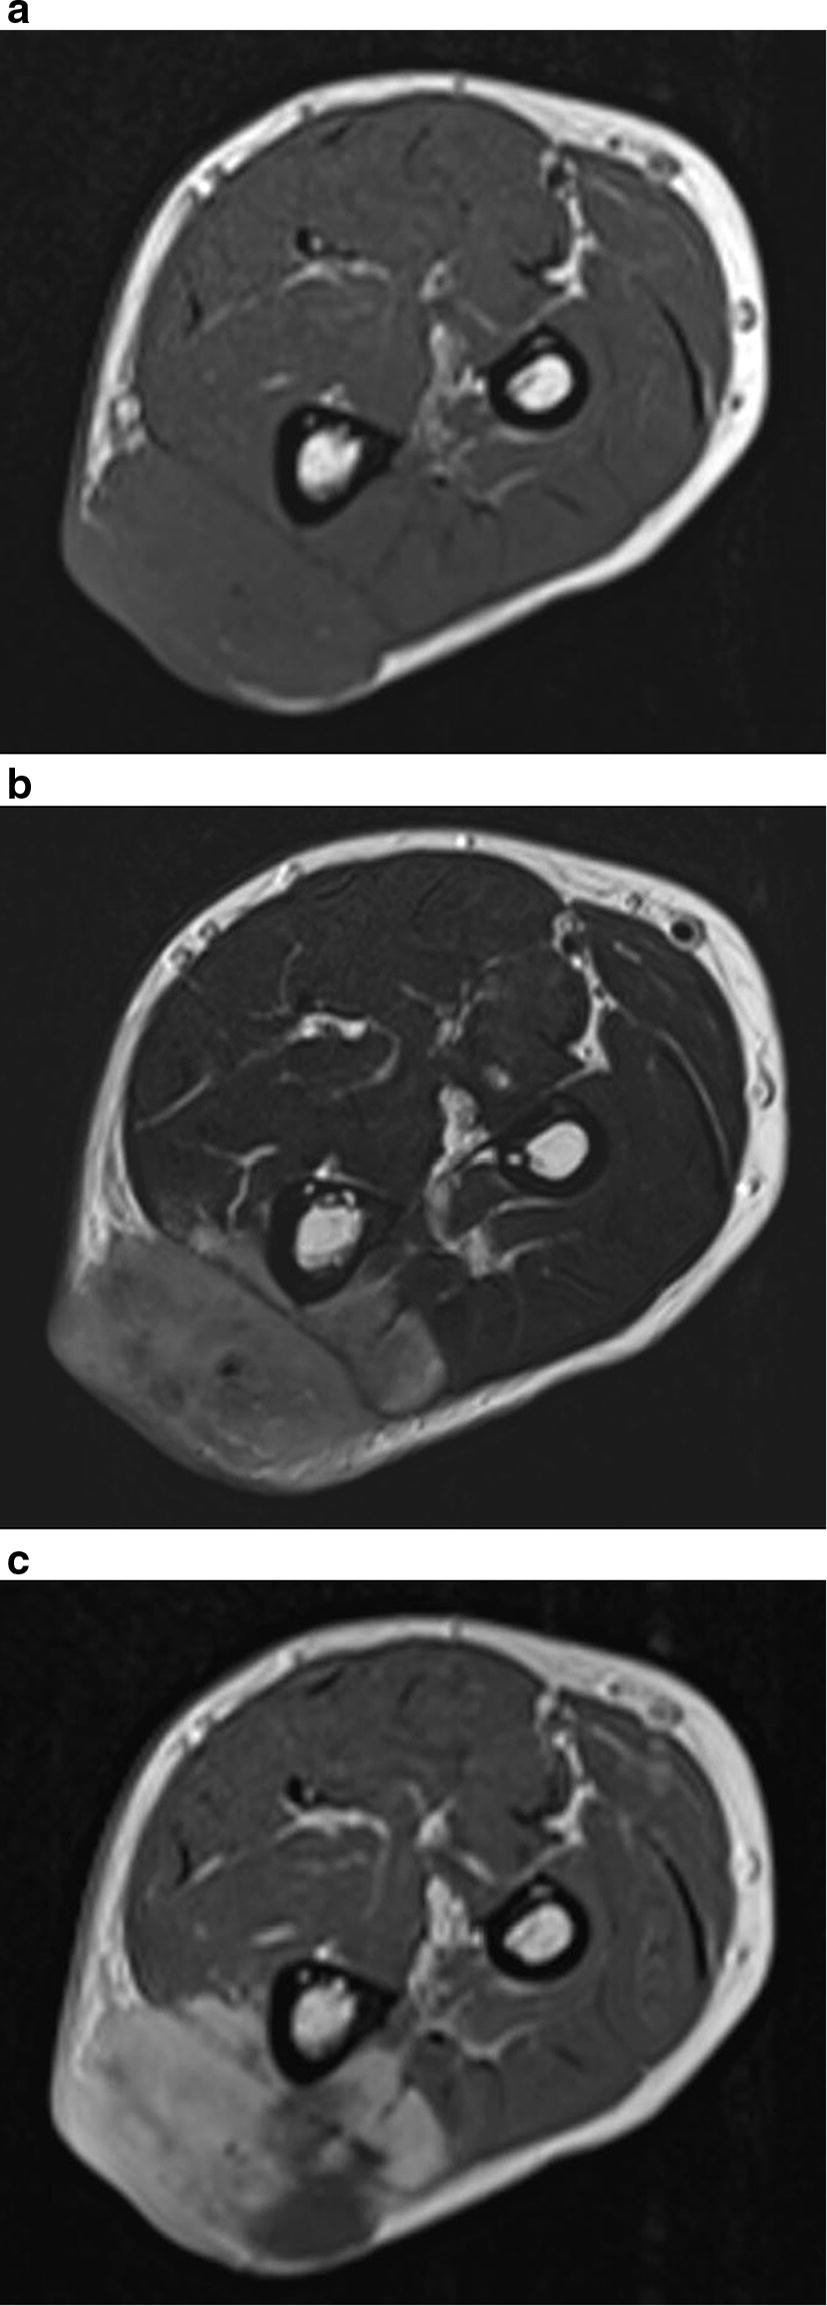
Magnetic Resonance Imaging (MRI) showing a soft tissue mass in the subcutis. The lesion is isointense to muscle in T1-weighted sequence (a), hyperintense on T2-weighted sequence (b), and shows contrast medium enhancement on T1-weighted sequence (c)
